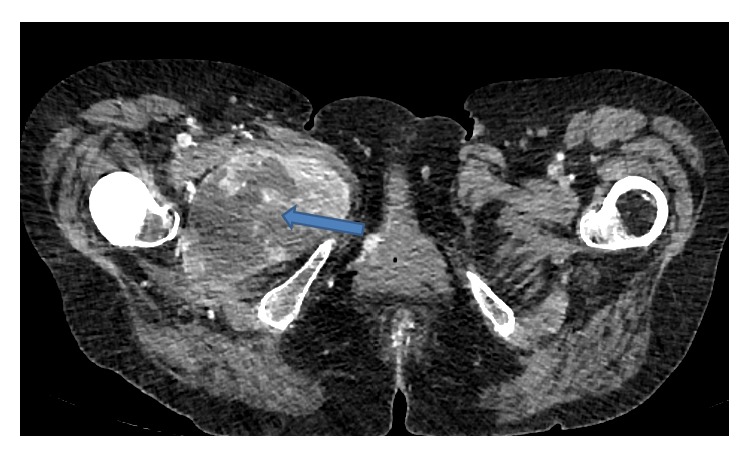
CT scan of the pelvis showing a cystic lesion in close proximity to the femoral stem suggesting an inflammatory pathology.
